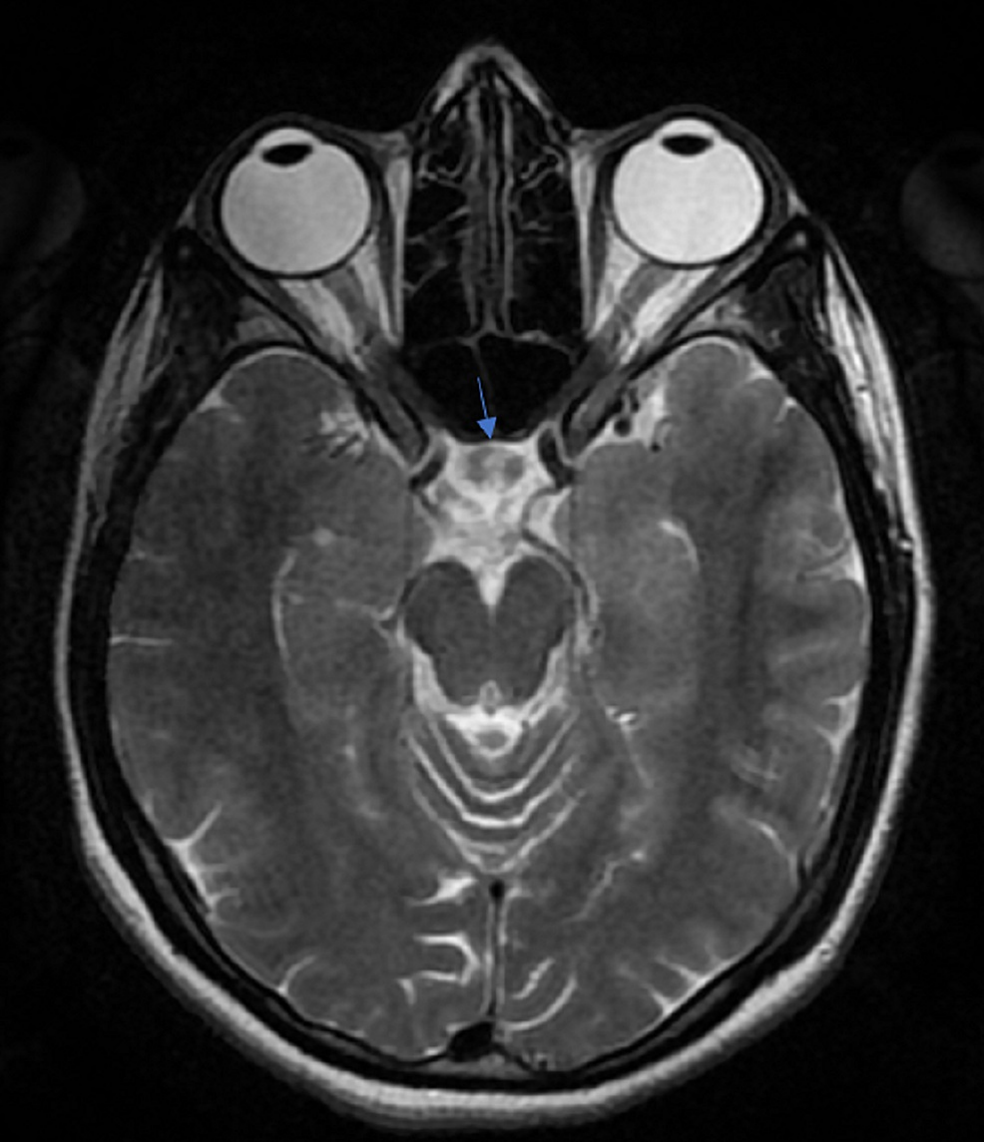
T2-weighted axial image showing two hypointense lesions, with hyperintense normal pituitary tissue between them (arrow).
